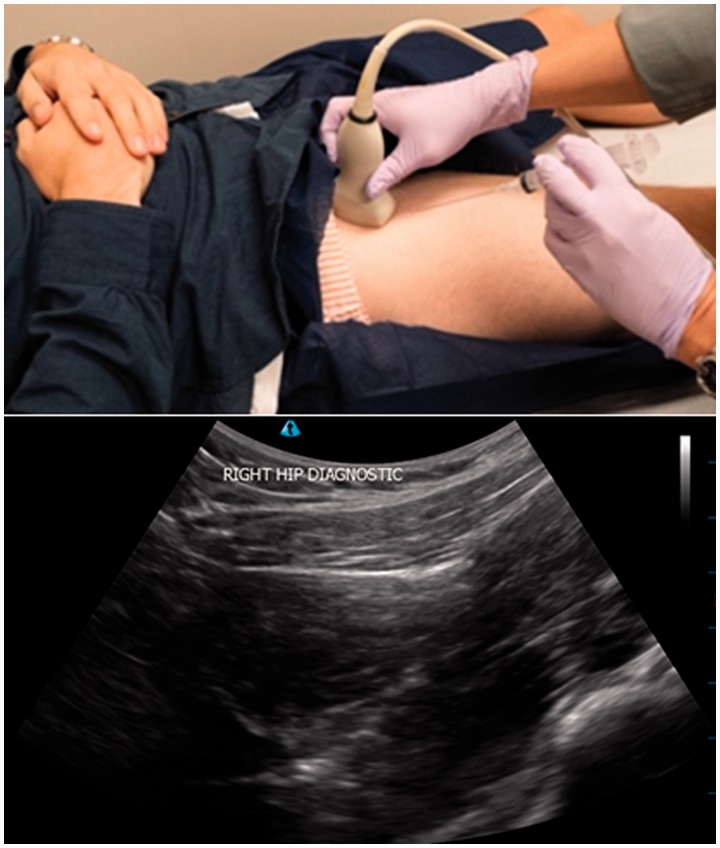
Protocol for intra-articular local anaesthetic injection for the hip: 10 ml of 1% lignocaine is inserted under ultrasound guidance. The patient is reviewed after 30 min for improvement of pain and impingement signs.
